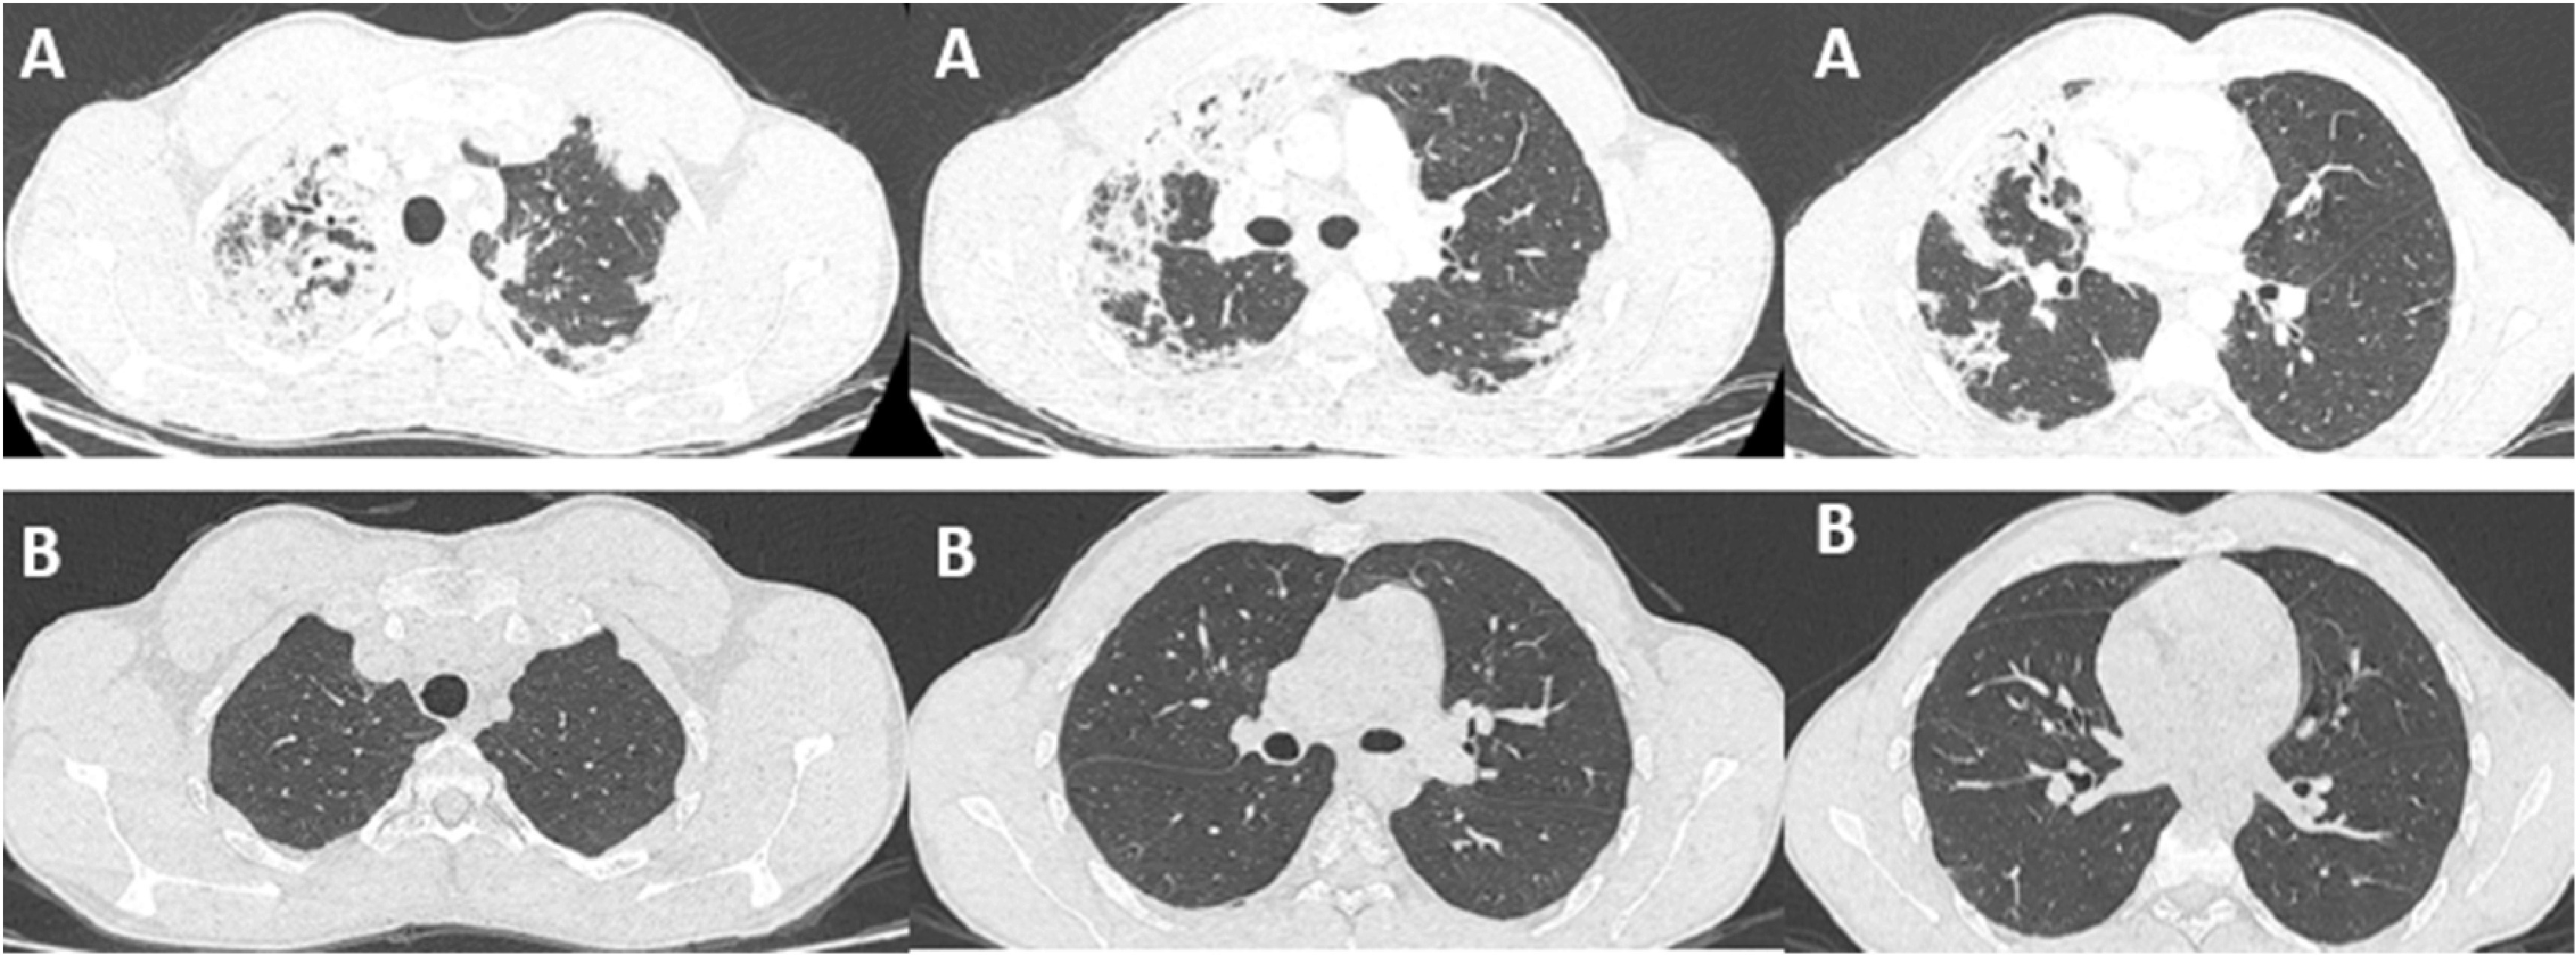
(A) CT chest of the first patient revealing bilateral and peripheral pulmonary infiltrates at the time of diagnosis. (B) CT chest of the first patient after 12 months of mepolizumab and tapering of corticosteroid dose, showing complete resolution of the previous infiltrates.
